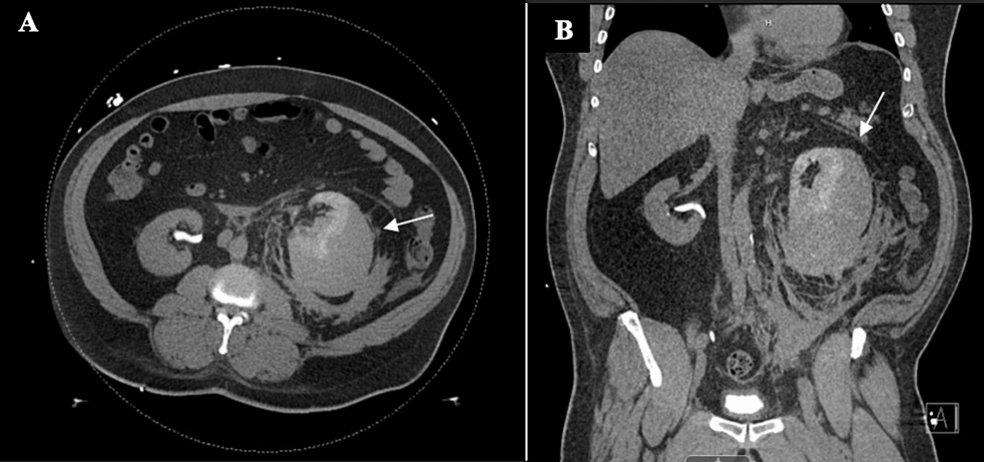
CT of the abdomen and pelvis without contrast. An enlarged left kidney and perinephric inflammation with subcapsular hematoma formation in the axial (a) and coronal (b) planes.
